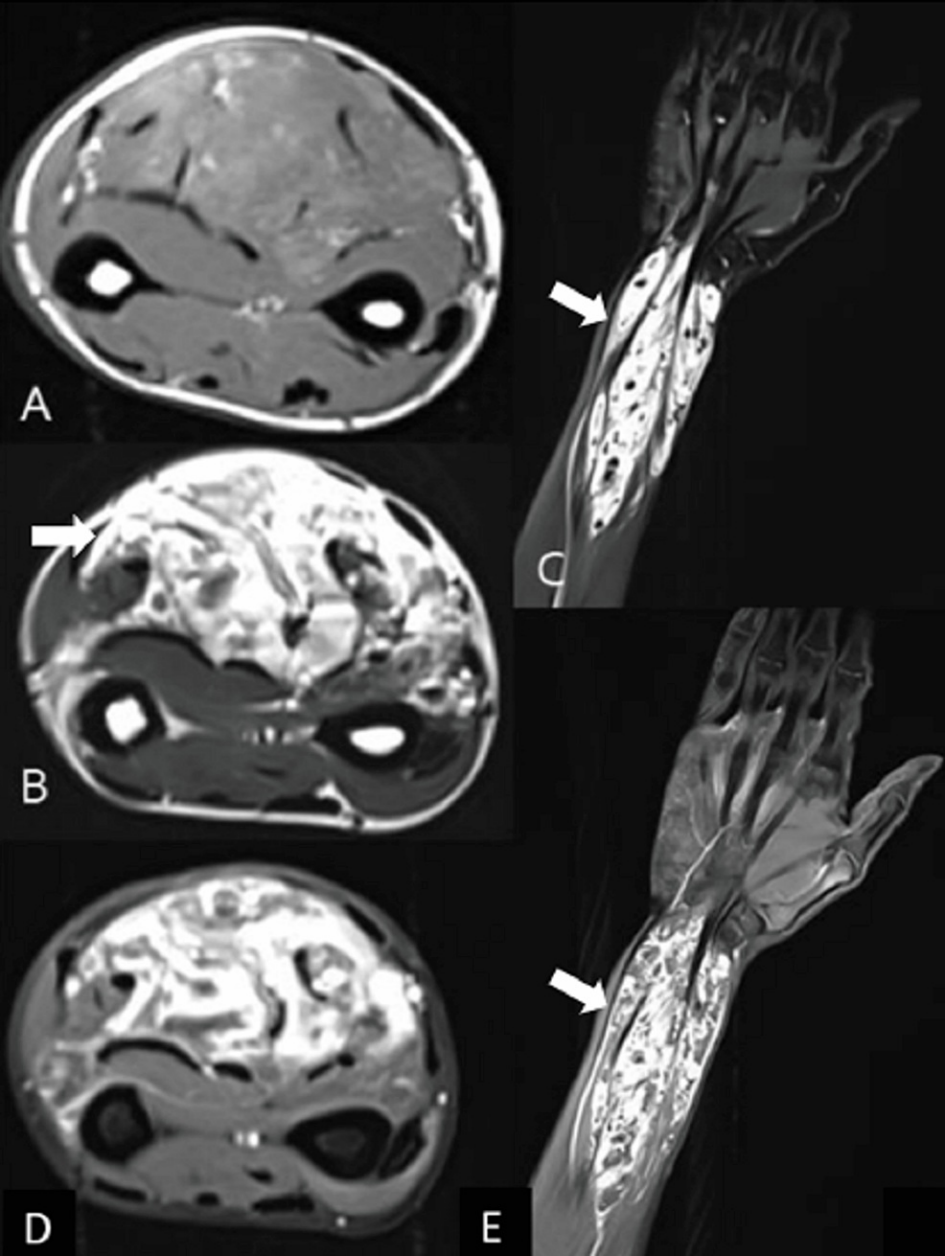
MRI of the forearm showing slow-flow venous malformation MRI of the right forearm in a 26-year-old female with progressively increasing swelling over the forearm for 10 years showing a large lobulated septated mass lesion seen in the subcutaneous, intermuscular, and intramuscular plane of the right forearm. The lesion appears heterogeneously iso-hyperintense to muscles on T1-weighted image (A), heterogeneously hyperintense on T2-weighted image (B) and short tau inversion recovery (STIR) (C, D) images, and shows heterogenous post-contrast enhancement with no enlarged arterial feeders or early draining veins (E). Findings are suggestive of slow-flow venous malformation.
